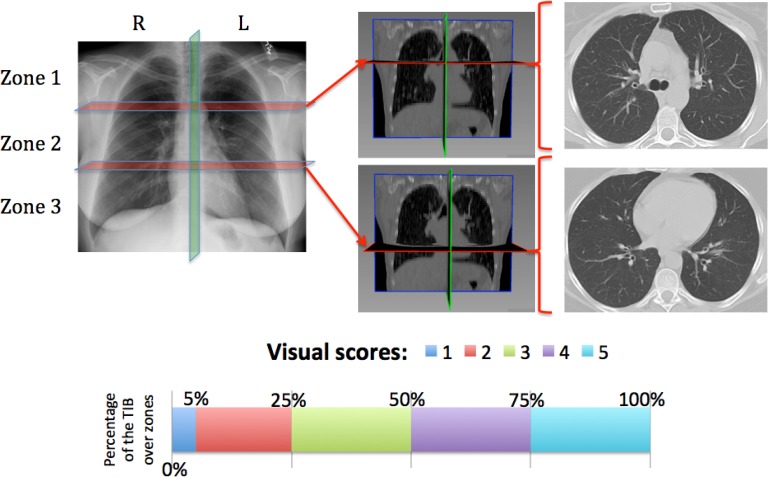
First row: lungs are divided into (left) three zones. Rough anatomical locations separating zones are shown in (middle) coronal and (right) axial CT slices, respectively. Second row: visual grading scheme.
